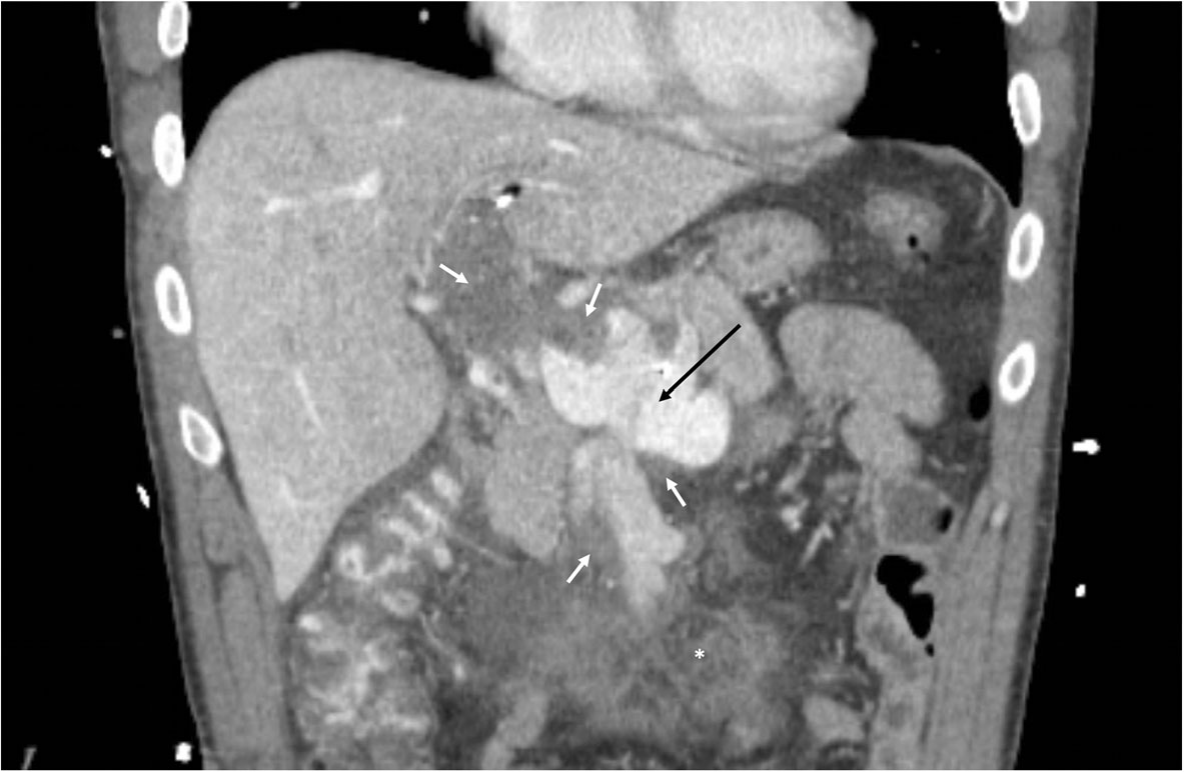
Coronal contrast-enhanced CT after thrombolysis and rheolytic thrombectomy. Although improvement is seen at the portosplenic confluence (black arrow), a large clot burden remains (white arrows). A transhepatic vascular sheath and thrombolysis catheter are partially visualized. Mesenteric edema (*) is also noted
